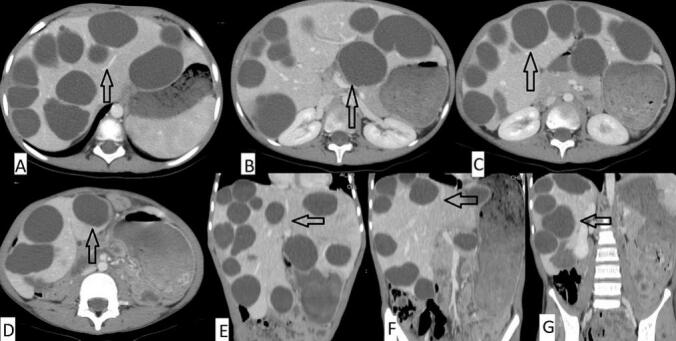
Presents axial CT images (A-D) and coronal (*E*-G) revealed an enlarged liver measuring 16 cm with multiple hypodense non-enhancing cystic lesions with thin enhancing walls in both lobes marked by black arrows, classified as 1CE. The largest hepatic cyst in segment II measured approximately 5.5 × 3.5 cm.
